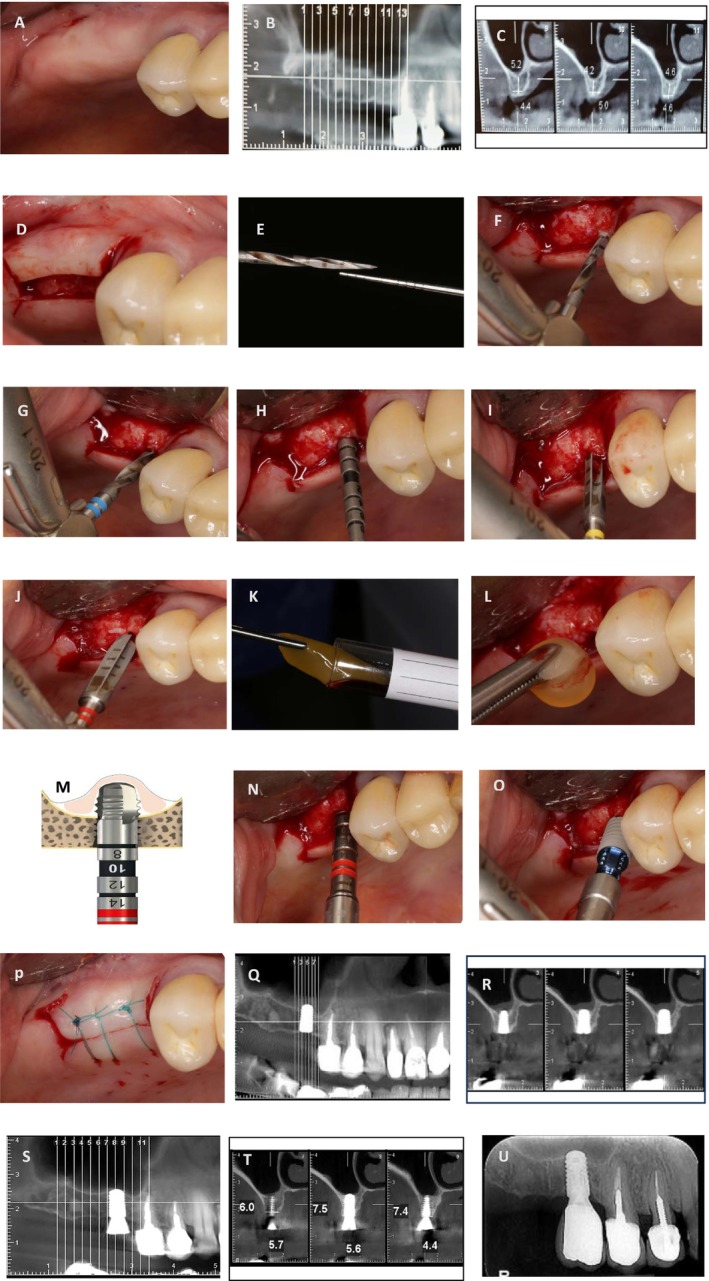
Case 1. (A) Pre‐operation view. (B, C) The ideal position of the implant. (D) Mid‐crestal incision. (E, F) The penetration depth of the starter drill within the close distance of the cortical bone of the sinus floor. (G) Insert a drill with a diameter of 2.2 mm, in the same manner as the starter drill. (H) Three‐dimensional positioning. (I) Inserting a 2.8‐mm‐diameter taper‐line drill based on RBH to perforate the cortical bone of the sinus floor. (J) Inserting a 3.5‐mm‐diameter taper‐line drill based on RBH to expand the cortical sinus floor hole. (K, L) Inserting PRF into the osteotomy hole. (M, N) The sinus membrane was elevated by transferring PRF using a taper bone tap drill into the sinus cavity. (O) Implant insertion (4.1 × 8 mm, BL). (P) Suturing (Nylon 5‐0). (Q, R) The CBCT view immediately following surgery. (S, T) The CBCT after 5 months. (U) The periapical view 1 year after the prosthetic delivery phase.
